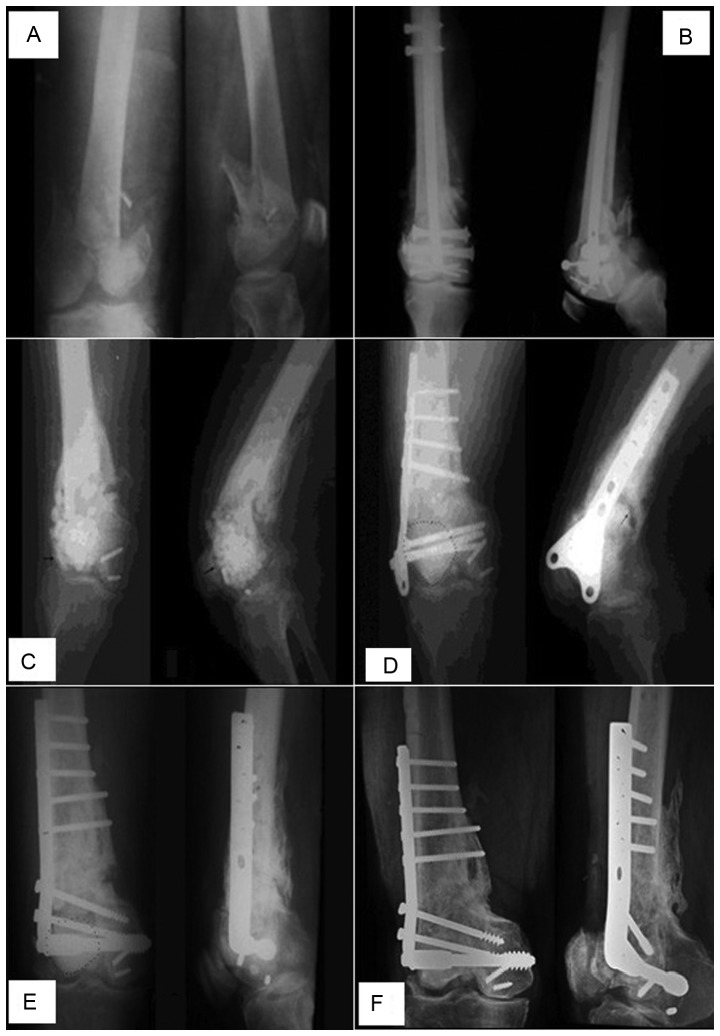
Postoperative infection of femoral condyle fracture. (A) Preoperative radiograph; (B) postoperative radiograph of internal fixation; (C) implantation of a vancomycin cement bead in the bone defect area (arrow) following debridement and dismantling of the internal fixation appliance; (D) implantation of a femoral condyle allograft from which the cartilage surface was removed (dotted line area) after the vancomycin cement bead had been removed and fixed by a strut plate; 8 months later, nonunion and displacement of the femoral condyle was observed (arrow indicates fracture line); (E) granulated cancellous autografting and a dynamic condylar screw plate was used to strengthen the fixation; this X-ray was produced 5 months after surgery (dotted line area indicates allograft); (F) 2 years after surgery, bone healing and existence of joint space was observed in the radiograph.
